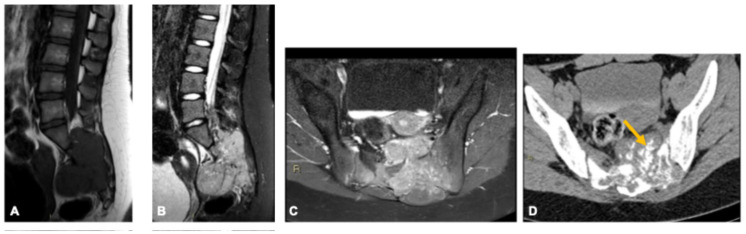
Osteosarcoma in a 9-year-old girl. Sagittal and axial MR images (A–C) demonstrate a large, eccentrically located lesion involving the left sacrum with cortical destruction, heterogeneous signal intensity, and extensive extraosseous soft-tissue extension. Post-contrast enhancement (C) is irregular, reflecting the tumor’s heterogeneous composition. Corresponding CT image (D) reveals mixed lytic and sclerotic changes with a dense, amorphous osteoid matrix (arrow).
